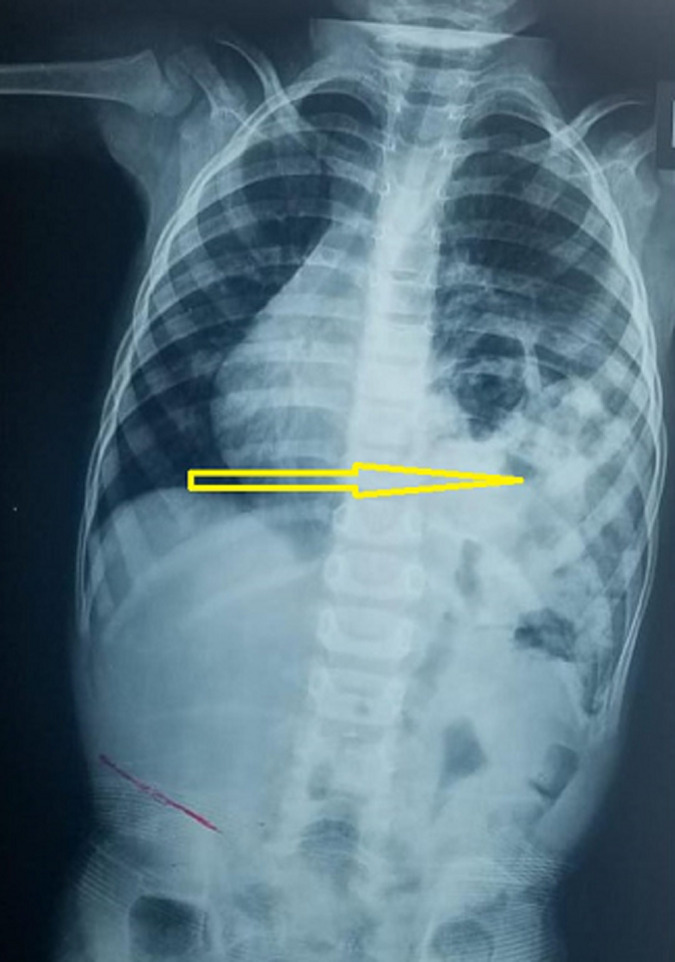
plain chest X-ray shows the left diaphragmatic hernia (arrow)
